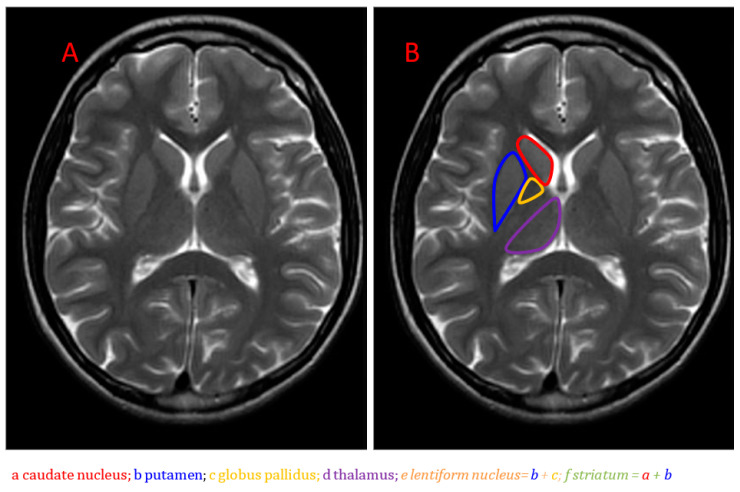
Brain anatomy on MRI, the basal ganglia on the T2-weighted image, axial plane (A,B).
